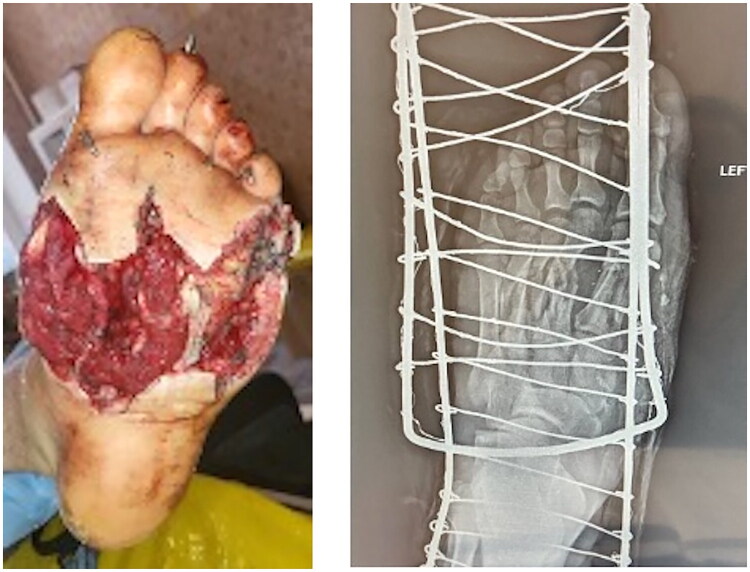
A - Clinical photograph on admission showing irregular 11x8 cm plantar defect with necrotic edges, exposed tendons, hyperemia and contamination; B - Plain X-ray demonstrating comminuted fractures of 1st–5th metatarsals stabilized with Kirschner wires, no major foreign bodies.
